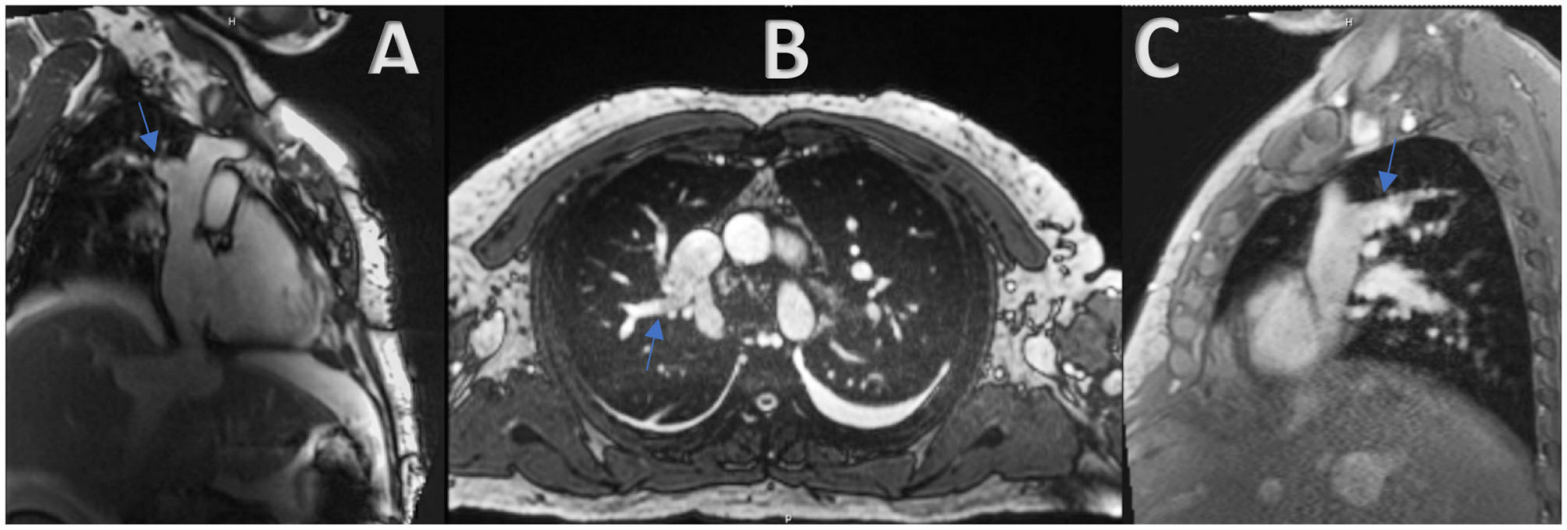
(A–C) Cardiac MRI revealing the presence of a large anomalous right upper lobe pulmonary vein draining into the upper SVC (Blue arrow).
